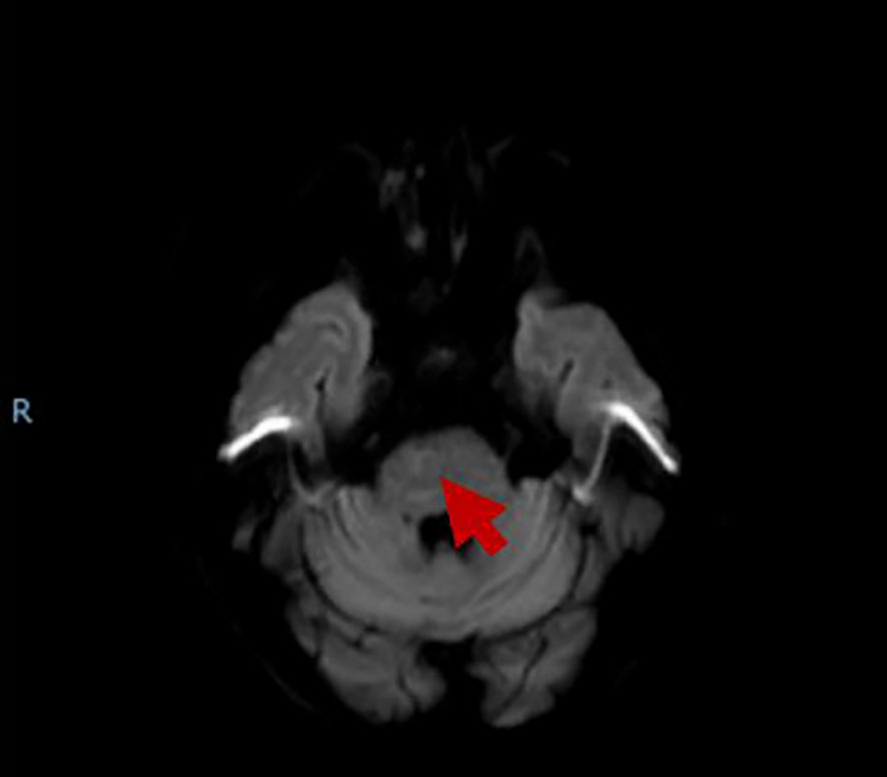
The MRI showed that brainstem infarction was subacute to chronic, and the restricted diffusion significantly reduced.
